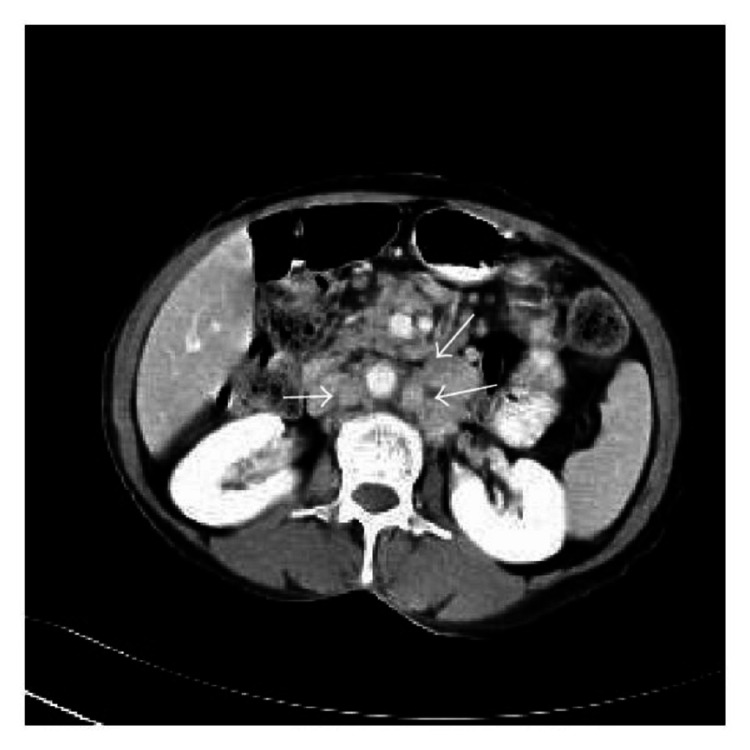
CT of abdomen revealing retroperitoneal lymphadenopathy.
